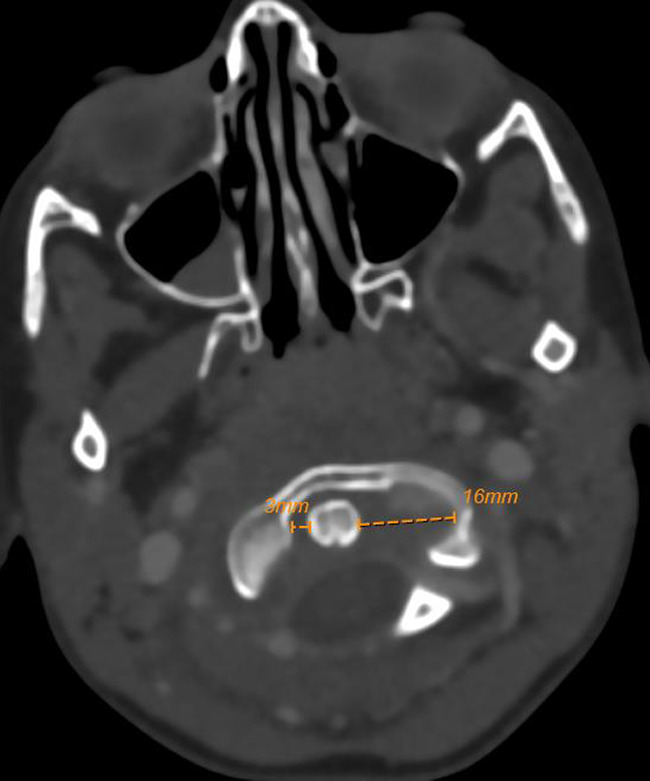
Cervical spine MRI showing mild rotatory atlantoaxial subluxation
